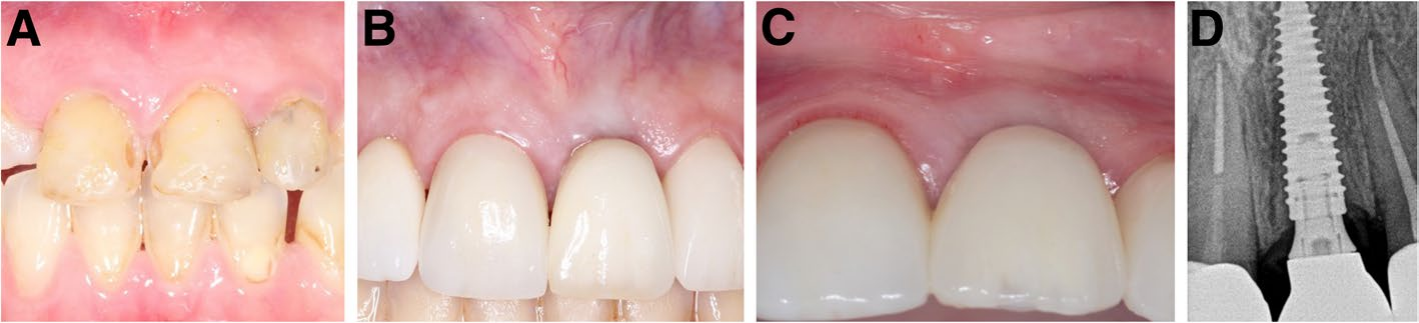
A-D: Photos and a radiograph of case #9 replacing the maxillary left central incisor. a Preoperative frontal view; b frontal view of the definitive crown at 12 months postloading; c incisal view of the definitive crown at 12 months postloading; and d periapical radiograph at 12 months postloading
